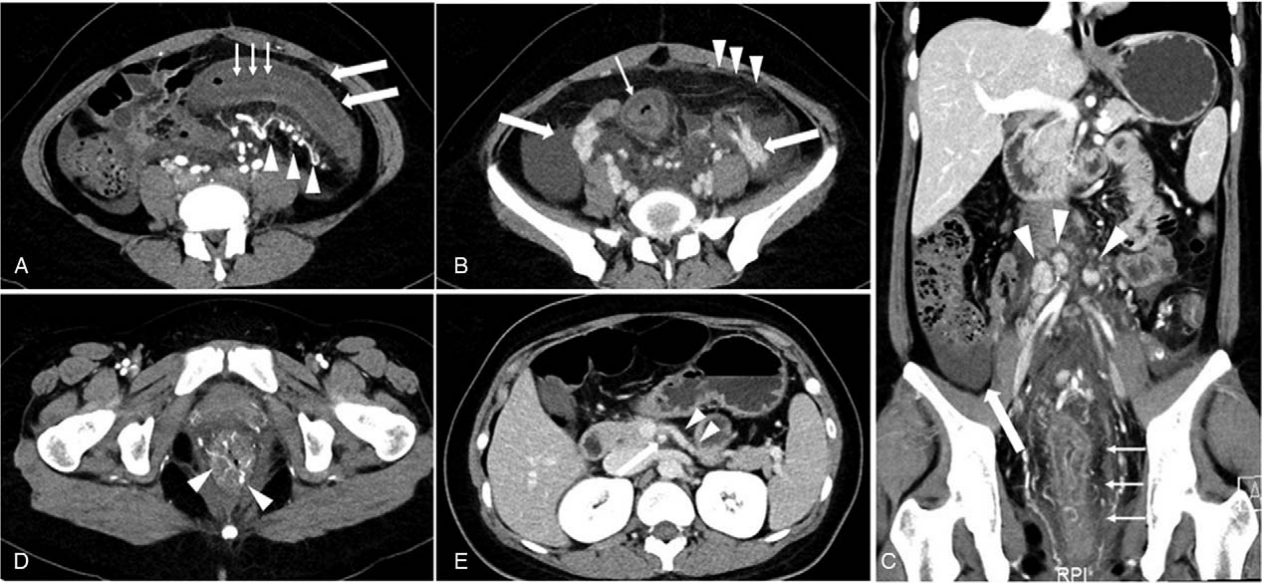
Abdominopelvic CT findings of the IMHMV. Axial image of arterial phase (A) demonstrated the continuous concentric thick, edematous colonic wall (thick arrows) with poor enhancement and ulceration (arrows) of colonic wall. The prominent pericolic arteries (arrowheads) with pseudoaneurysm are the specific signs of IMHMV. Axial image of portal phases (B) showed the fat stranding, omental cake sign (arrowheads), and dilated ovarian veins (thick arrows). Oblique coronal reformatted image (C) of portal phase demonstrated severe rectum wall thickening with mural stratification, pericolic fat infiltration (arrows) and ascites (thick arrows). Many retroperitoneal enlarged lymph nodes were showed (arrowheads). Pseudoaneurysm (D) in the submucosa might be responsible for the bloody diarrhea. Axial delayed phase image (E) demonstrated the patent inferior mesenteric vein (arrowheads), superior mesenteric artery and superior mesenteric vein (thick arrows). CT = computed tomography, IMHMV = idiopathic myointimal hyperplasia of mesenteric veins.
